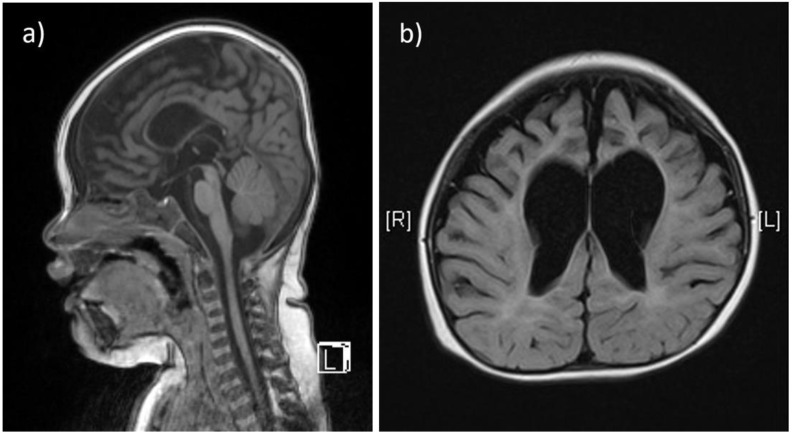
The T1-weighted, sagittal section of brain MRI from patient 1 shows thinning of the corpus callosum (a). The T2-weighted, axial section from the same patient shows diffuse cerebral atrophy involving both gray and white matters, and sulcal widening with prominent enlargement of the ventricular systems, especially at the frontal horns (b).
